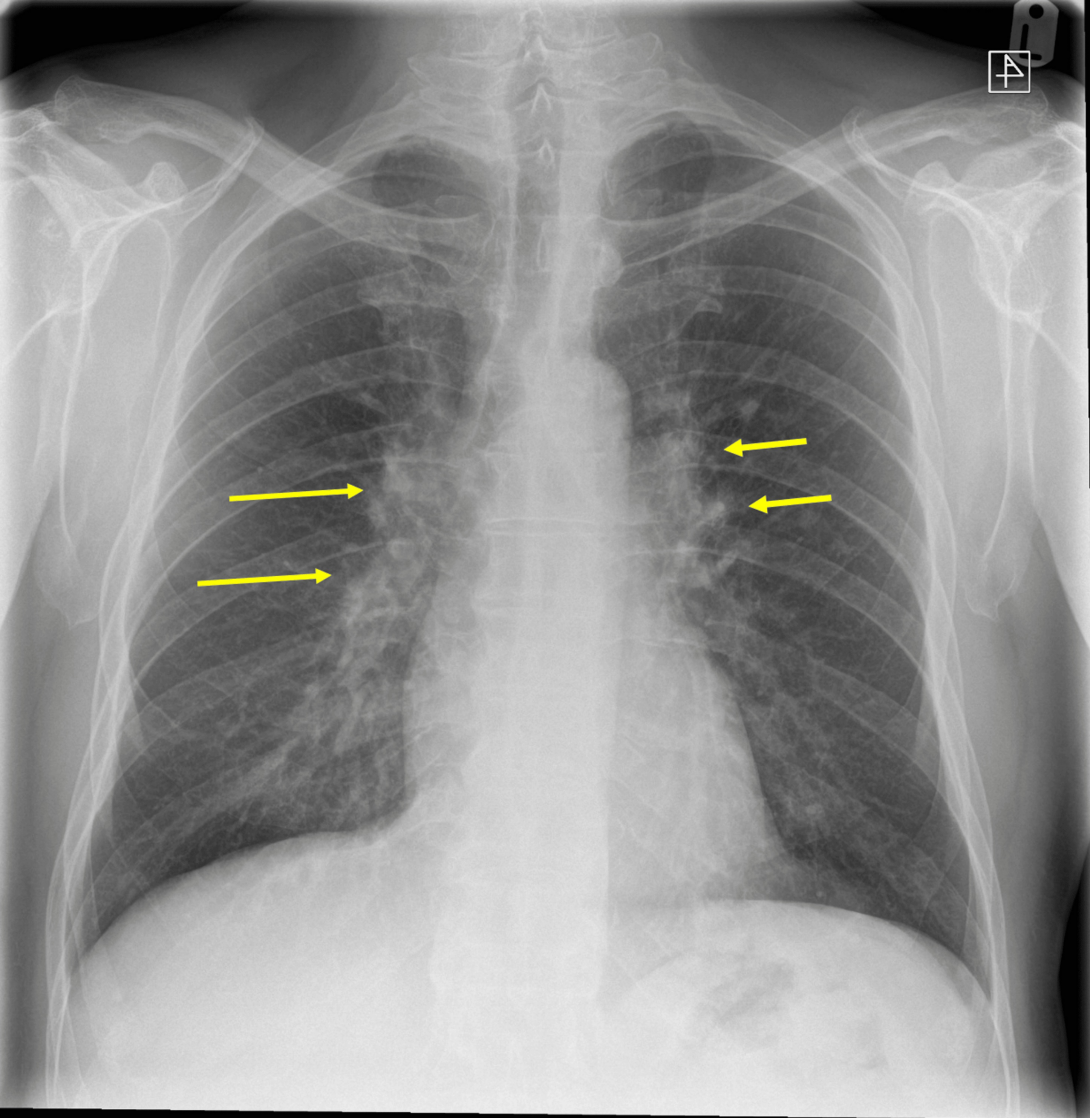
Frontal chest X-ray shows bilateral bulky hila along with small nodular opacities in the left mid zones (yellow arrows), suggesting lymphadenopathy and pulmonary involvement.
